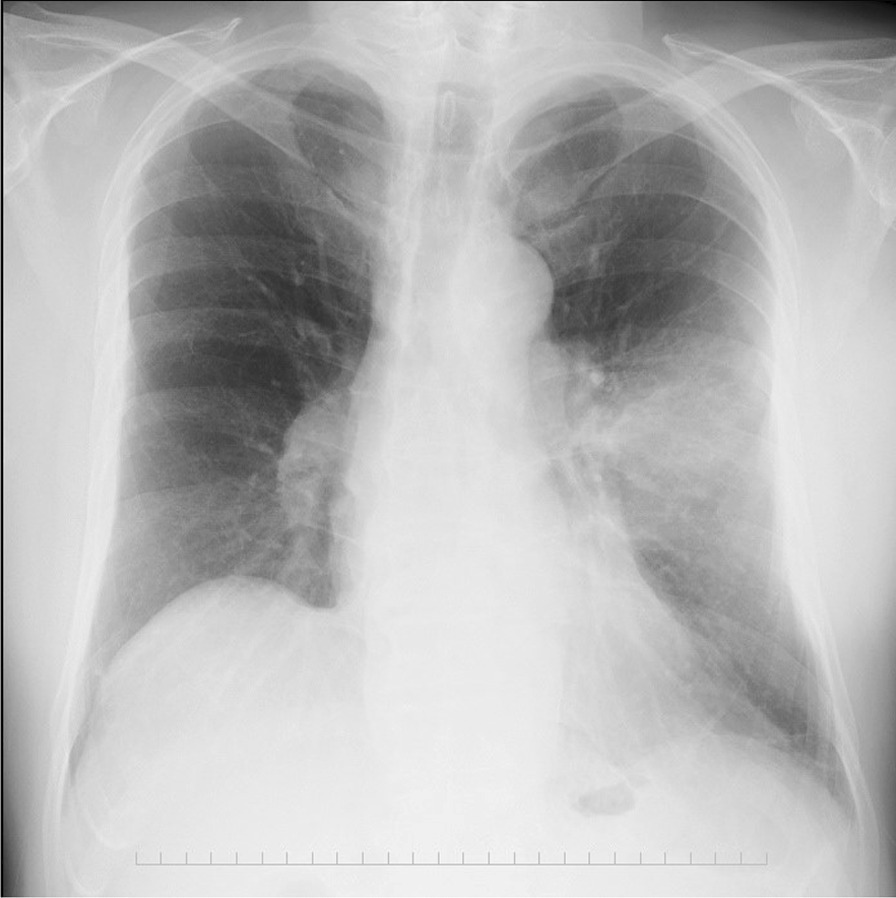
Chest radiography image upon presentation
